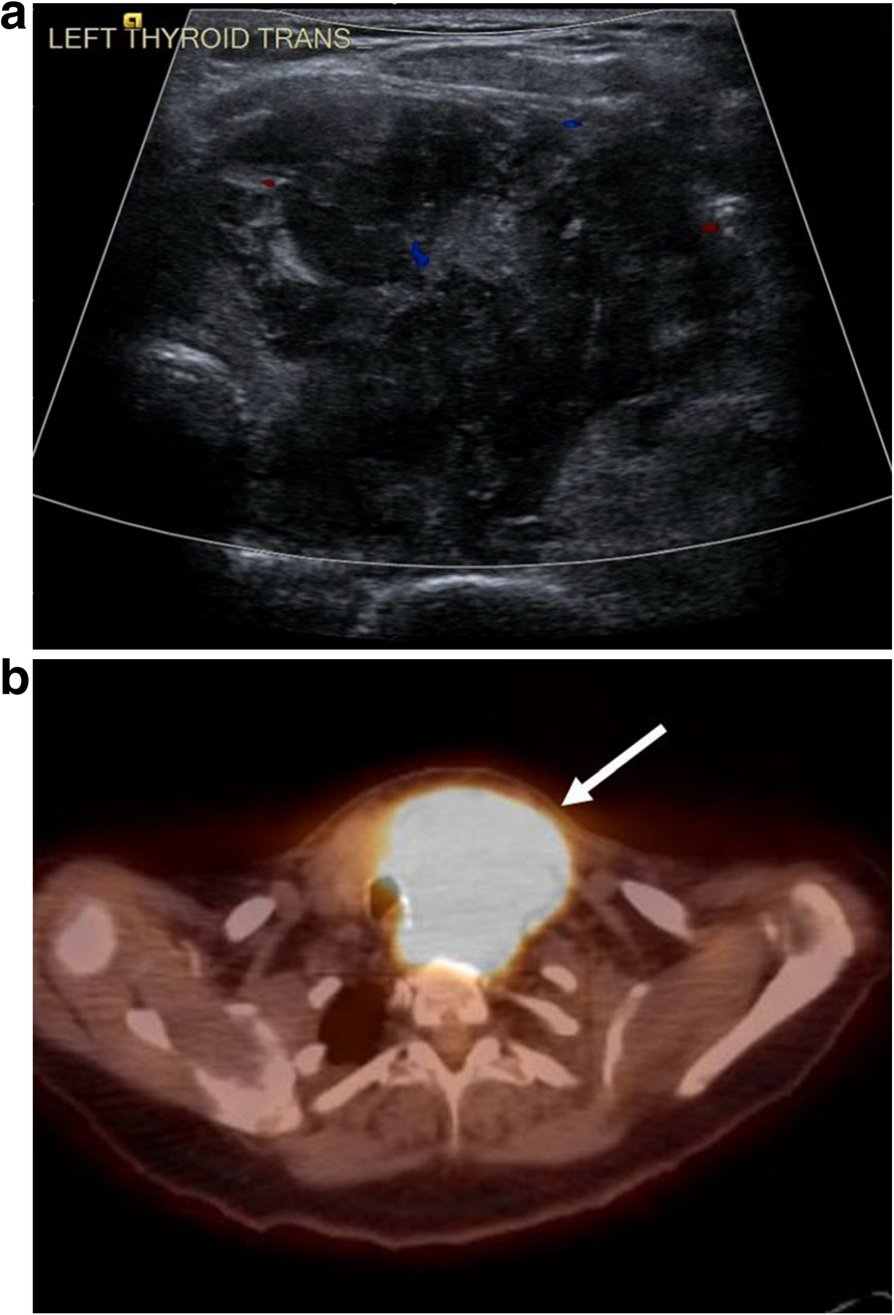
Thyroid involvement. A 58-year-old female presenting with dysphagia. a Transverse color Doppler image through the left thyroid demonstrates a large, heterogeneous hypovascular mass. b Fused axial PET-CT demonstrates the thyroid mass with rightward tracheal displacement and associated hypermetabolic activity. Biopsy of the thyroid mass revealed Burkitt lymphoma
